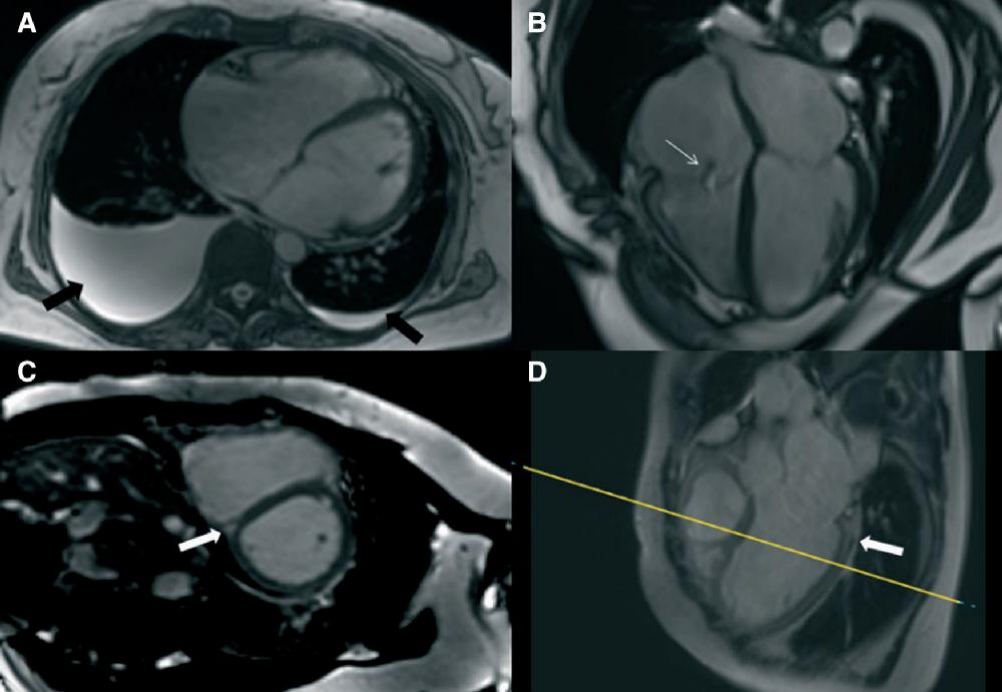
Cardiac magnetic resonance imaging: (*A*) bilateral hydrothorax; (*B*) dilated cardiac chambers, biventricular dysfunction: left ventricular ejection fraction 22%; right ventricular ejection fraction 32%; (*C*, *D*) signs of contrast material accumulation at the point of insertion of the ventricles.
